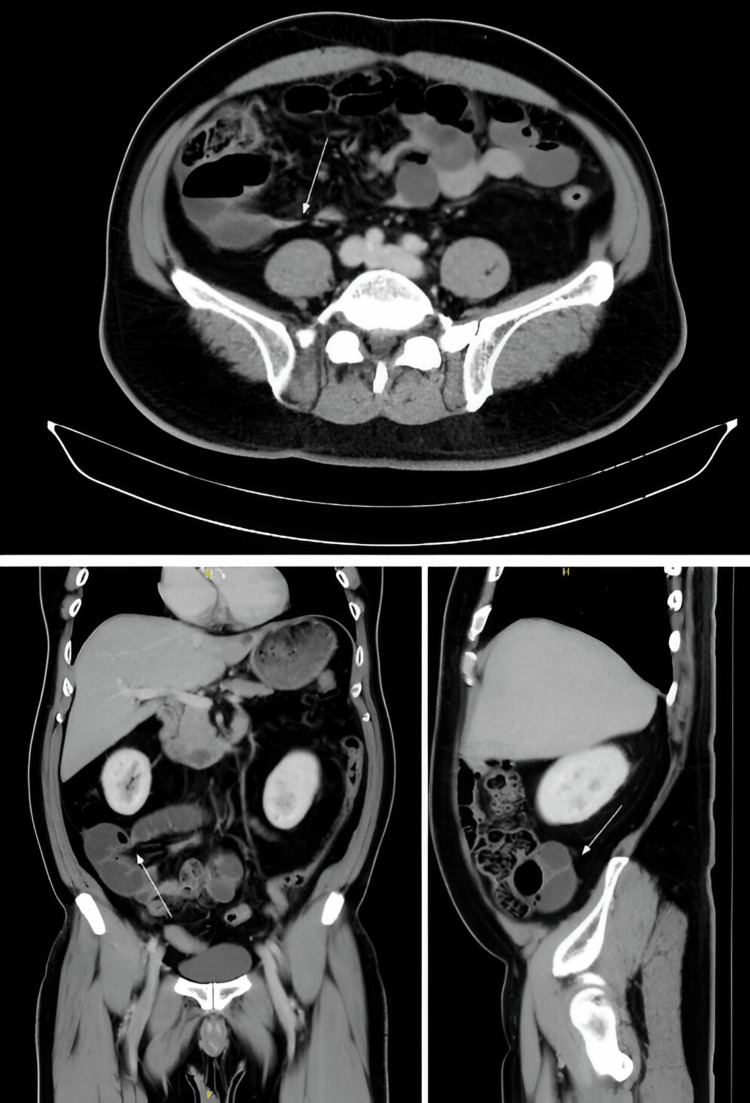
CT of the abdomen and pelvis. Axial and coronal images demonstrate sharp angulation of the small bowel loops (arrows) at the right iliac fossa possibly because of adhesion bands. Sagittal image demonstrates a loop of undilated small bowel posterior to the cecum (arrow) with preserved mural enhancement
